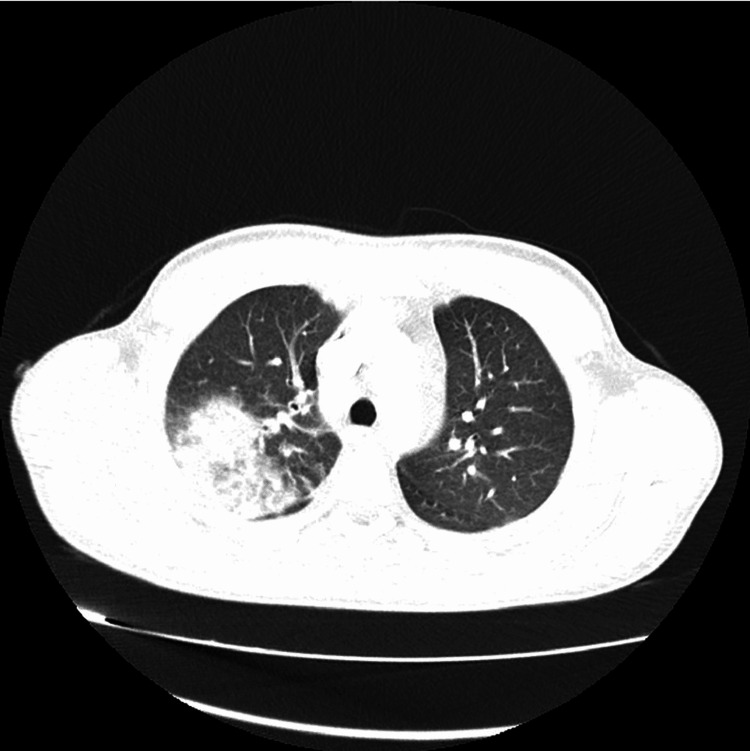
CT lung CT lung showing right-sided pneumonia and right-sided pleural effusion CT: computed tomography
